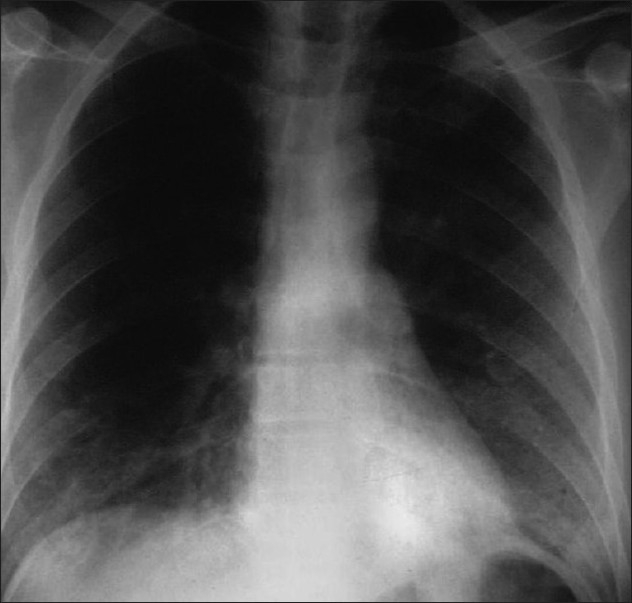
Pneumocystis carinii* pneumonia atypical features. Chest X-ray shows focal consolidation of the left lung base
